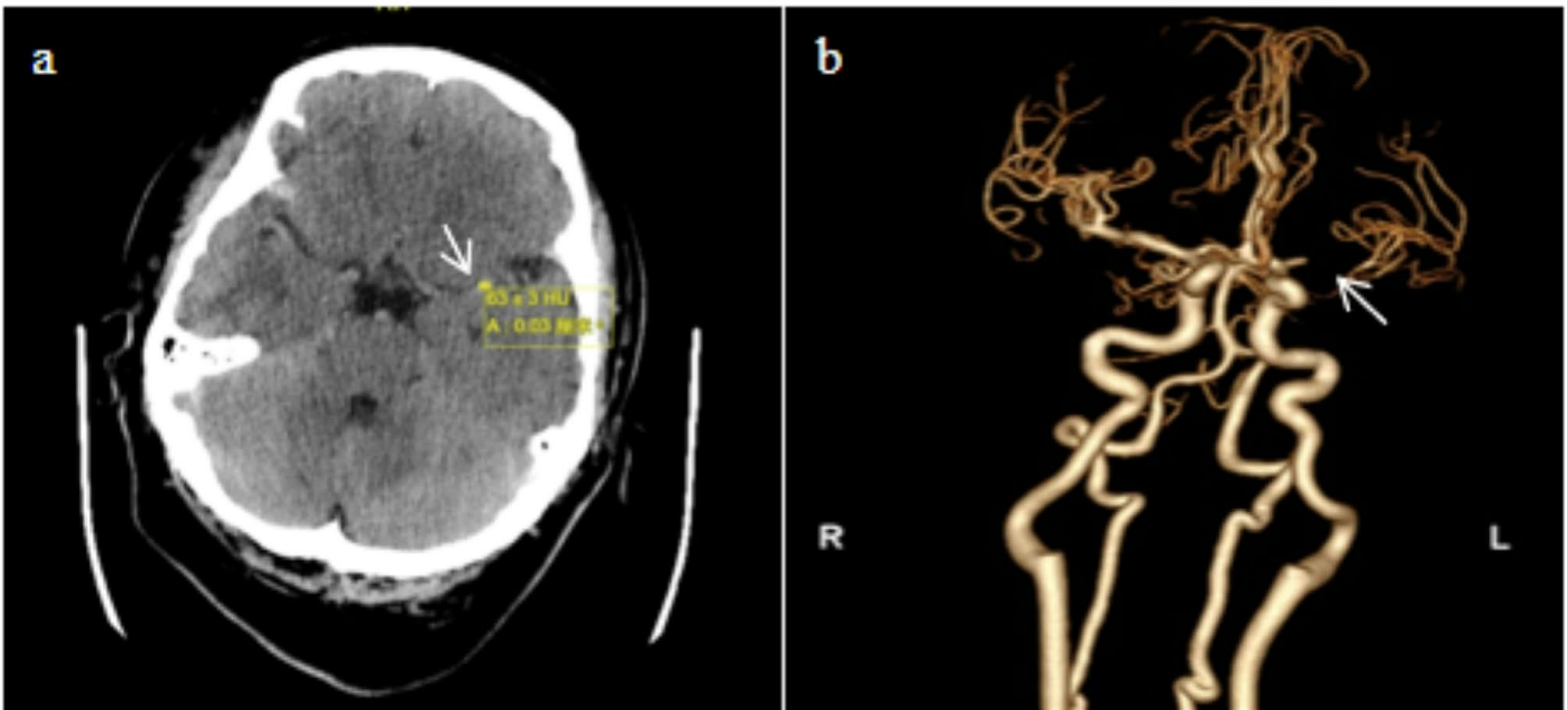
Preoperative imaging examination. aBrain computed tomography showed a high density of the left MCA. b Computed tomography angiography (CTA) showed a left M1 occlusion
